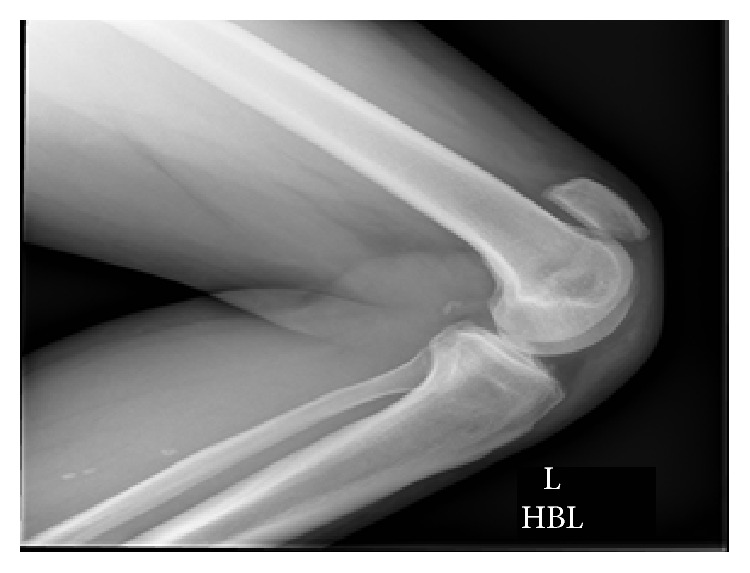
Lateral radiograph of left knee.
